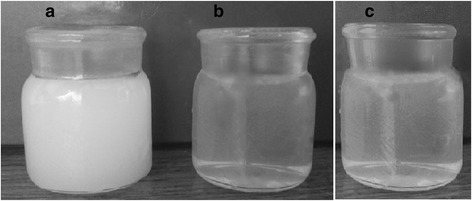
Photographs of the nanocellulose vials prepared after hydrolysis of OSP by sulfuric acid of concentration 43% at 60 °C and 60 min: without ultrasound treatment (a) and after ultrasound treatment (b), and after 3 months of storage (c)
